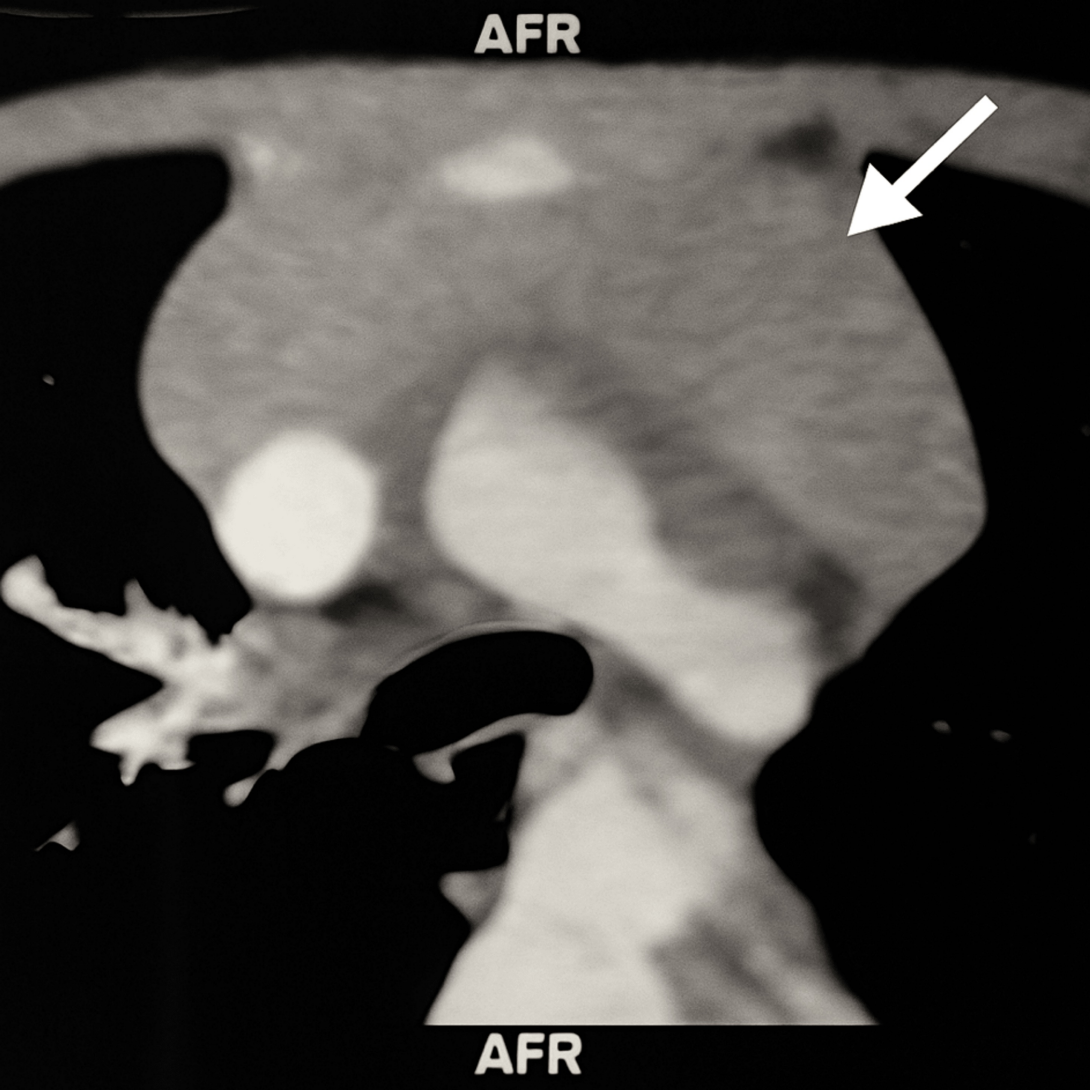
Chest CT scan showing an anterior-superior mediastinal soft tissue mass measuring 74 × 24 mm (white arrow) CT: computed tomography
